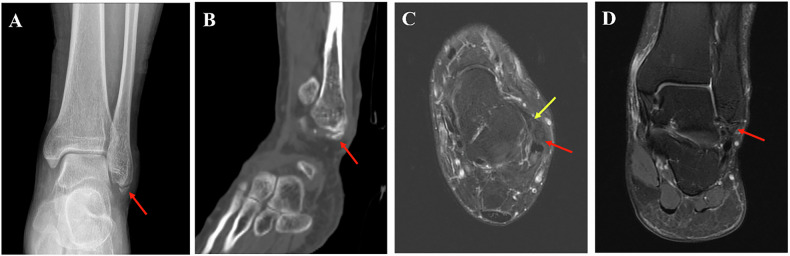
Imaging findings in a 41-year-old male NAF patient. A Ankle anteroposterior radiograph: the SFO (red arrow) exhibits a congruent relationship with the lateral malleolus. B Sagittal CT reconstruction (bone window): the SFO (red arrow) demonstrates an irregular contour, cortical irregularity, and an anteroposterior long-axis orientation. C Axial PDWI: the SFO (red arrow) is located anteromedial to the lateral malleolus and exhibits continuity with ATFL (yellow arrow). D Coronal PDWI: the SFO (red arrow) displays an absence of T2-hyperintense signal between it and the fibula, and the ossicle itself shows no evidence of associated marrow edema
